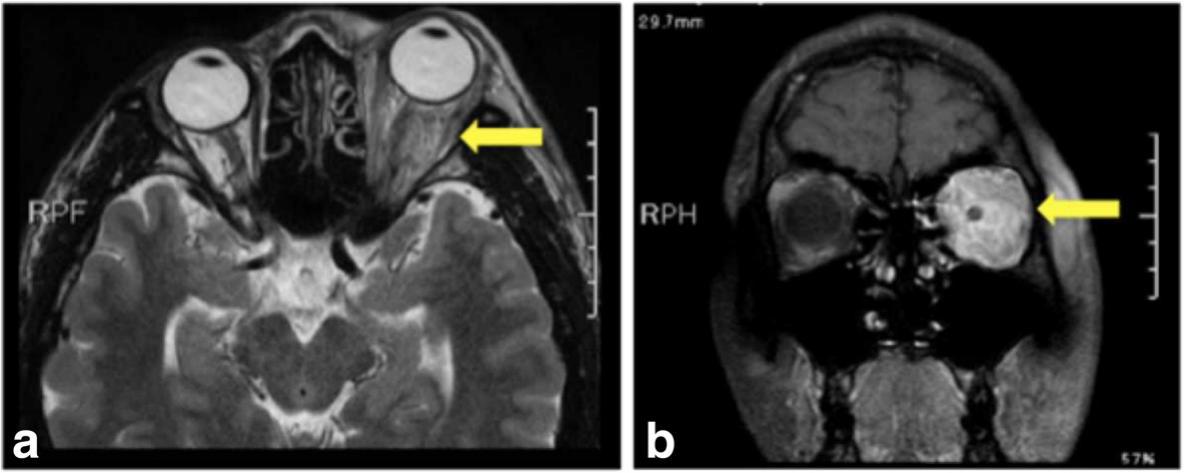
Orbitographic magnetic resonance imaging and a T_2_-weighted image from the first visit. a A systematic trachychromatic image of the left periorbital soft tissues and (b) soft tissue in the left orbital muscle cone is swollen, and the left eyeball is protruding
